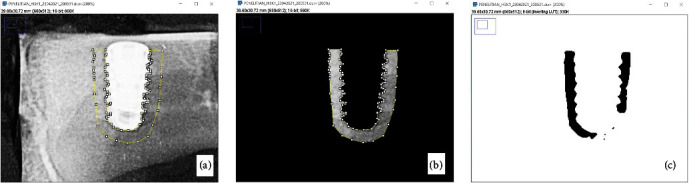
The density analysis and bone morphometry imaging process: (a) ROI selection on periapical radiographs; (b, c) digital processing on ImageJ cropping-filter Gaussian blur-thresholding.
